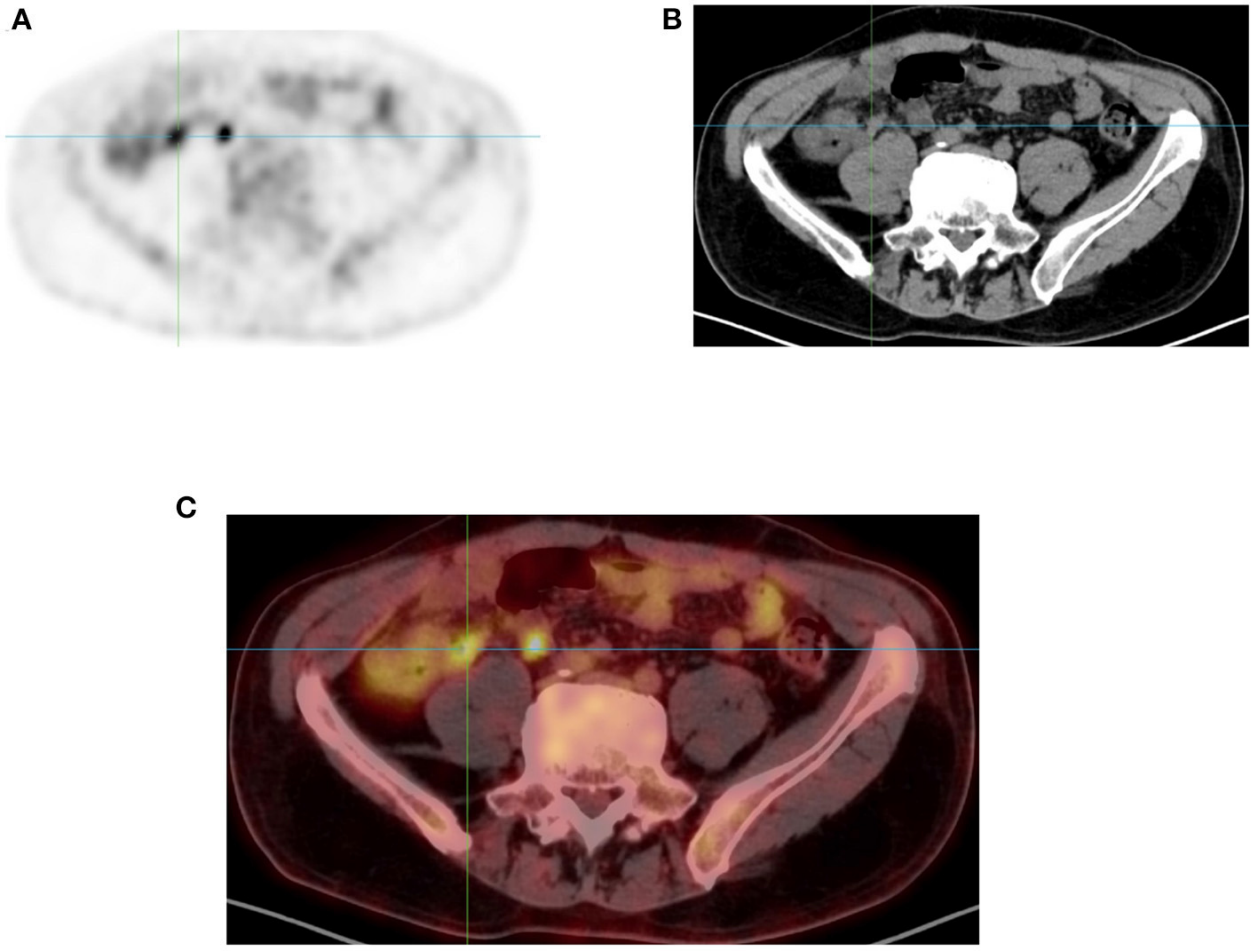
Appendix uptake. FDG PET Axial image (A) showing linear increased uptake in right iliac fossa that corresponds to appendix as correlated in anatomical CT (B) and combined PET/CT (C) images of the same plane (see crosshair in each image).
